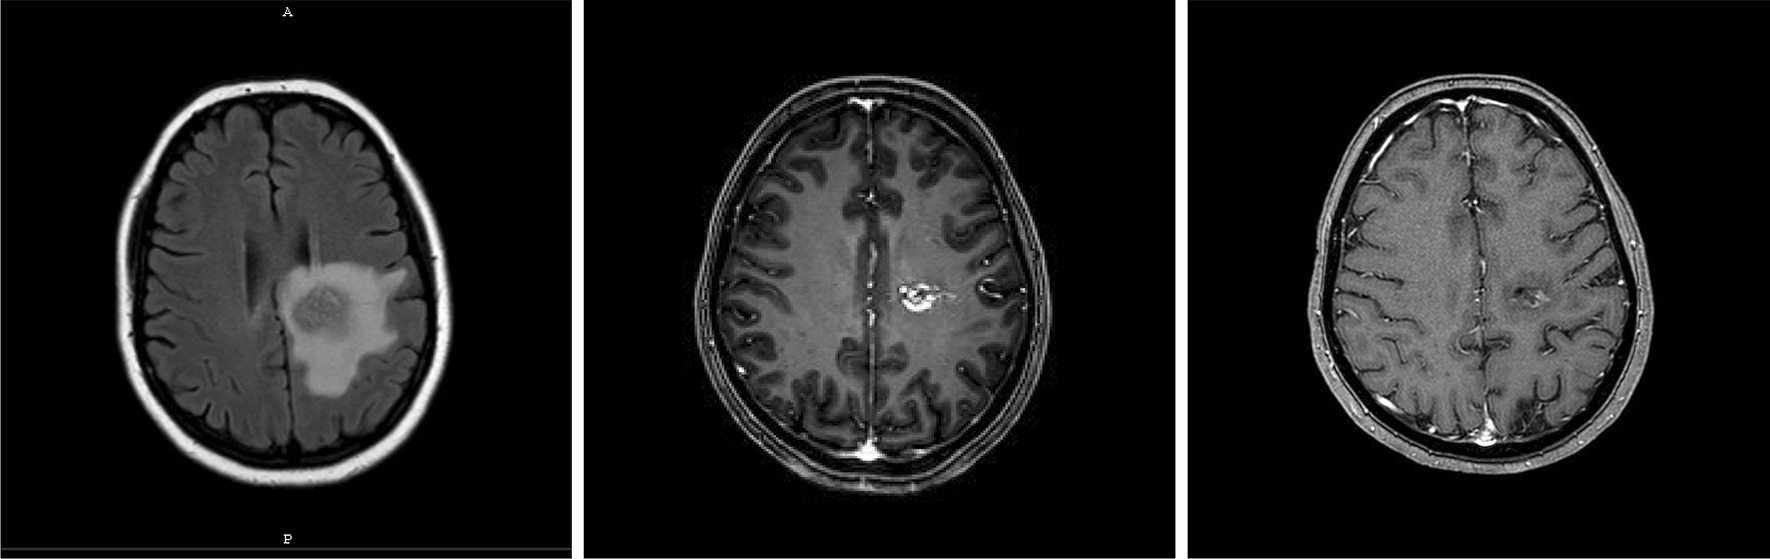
Post-SRS follow up (1) MRI shows post-SRS oedema (2) Partial obliteration (3) complete obliteration
